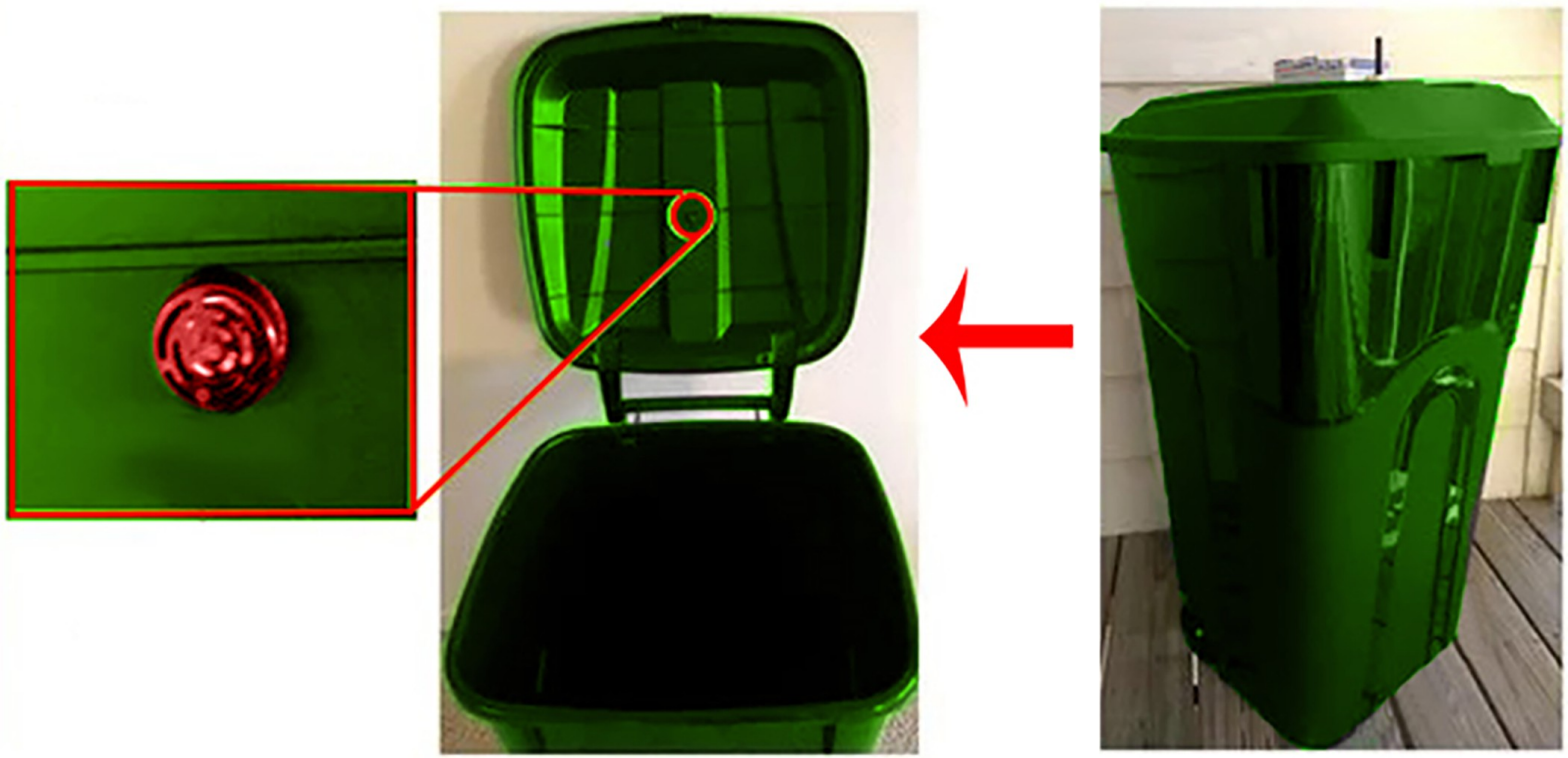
(a) Smart waste bin equipped with ultrasonic sensor, (b) smart waste bin equipped with IoT device.
